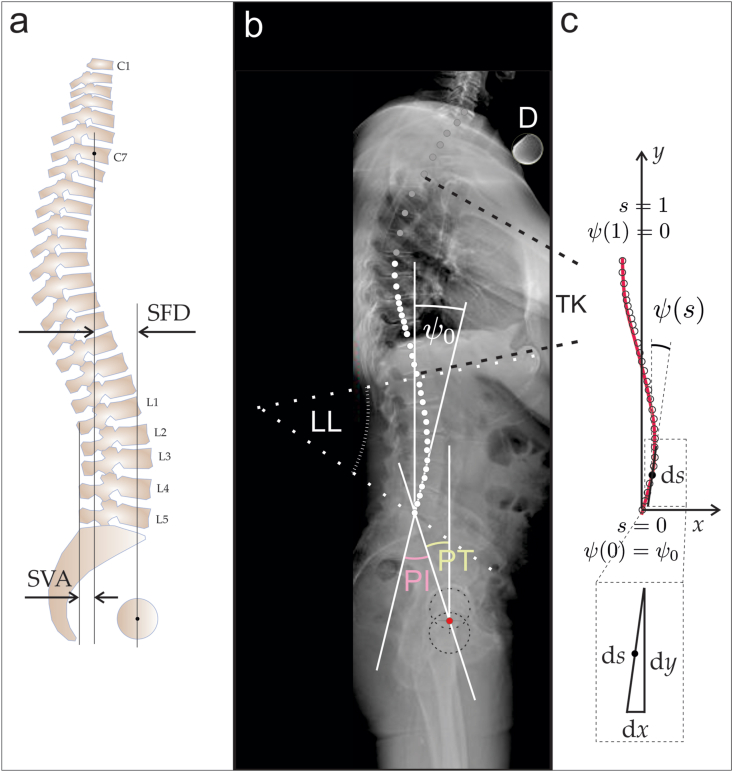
a: Scheme of the spine with parameters SVA and SFD which determine Sagittal Balance SB = SVA/SFD; C7 marks the 7th cervical vertebra and L1-L5 mark the lumbar vertebrae; b: A side radiograph of the spine in a two-legged stance with geometrical parameters: lumbar lordosis (LL), pelvic incidence (PI), pelvic tilt (PT) and sacral slope ψ_0_; D indicates a unit sphere with known diameter and TK indicates Thoracic Kyphosis; c: Shape of the spine assessed from an example radiograph (white dots), theoretically predicted shape of the spine (red line), and parametrization of the spine model curve by two dimensionless coordinates (*x* and *y*).
